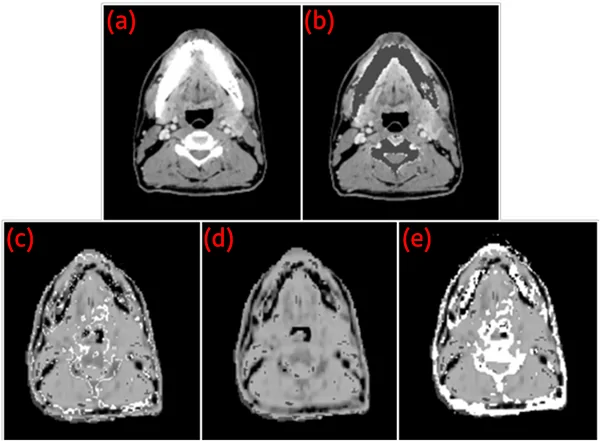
Slice of the volume of the same patient for CT (a), CT without bone (b), DA sCT (c), DA sCT without bone pixels (d), and DA sCT with bone overestimated (e).
